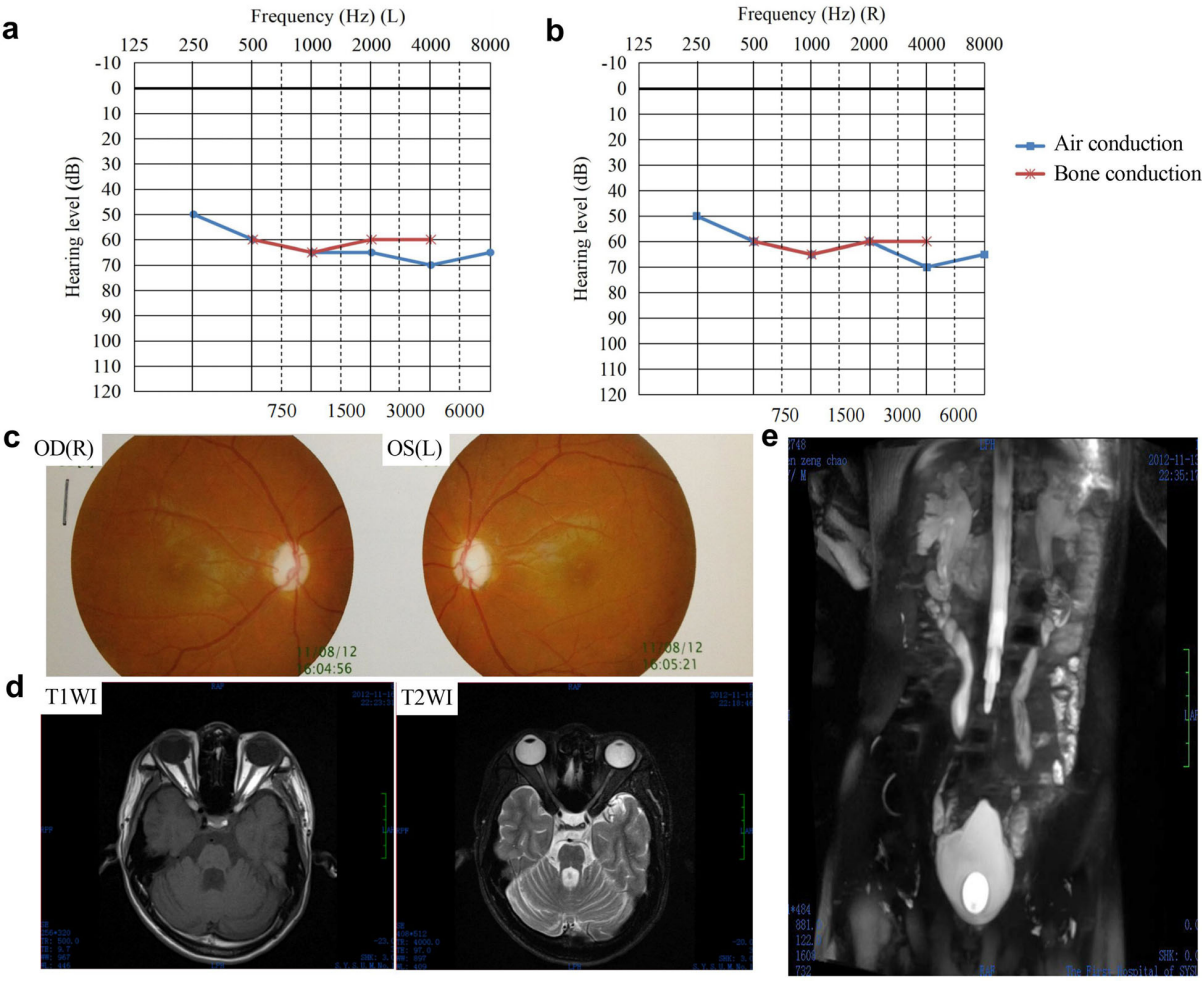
The patient presented with hearing loss, decreased vision, optic atrophy and neurogenic bladder. a Audiograms show left sensorineural hearing loss. b Audiograms show right sensorineural hearing loss. c Fundus image shows atrophic optic discs with temporal pallor. d Axial brain MRI shows bilateral optic nerve atrophy on T1WI (left) and T2WI (right). e MRU shows marked dilation of bilateral renal pelvis, calyces and ureter
